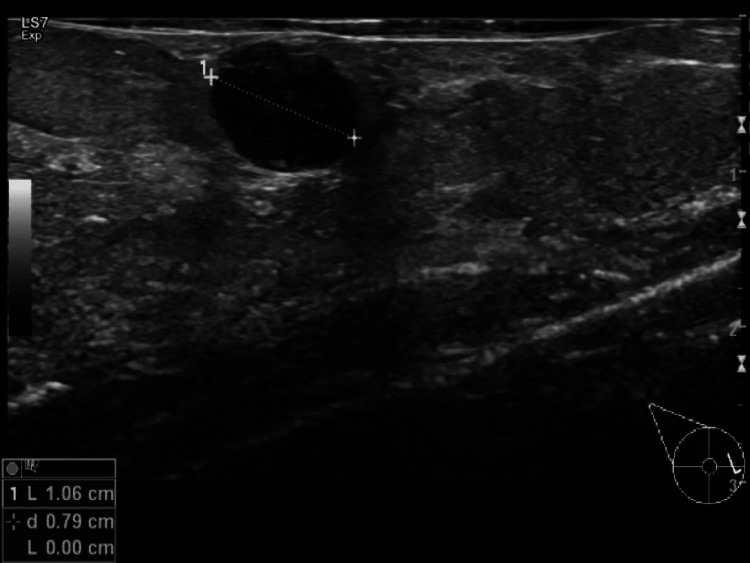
Case three ultrasound showing a nodular lesion in the inner quadrants.
